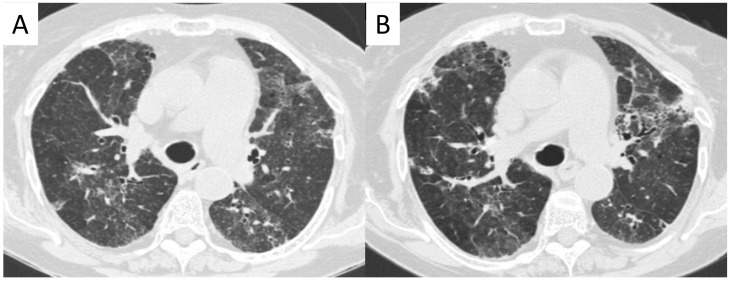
An 80-year-old female patient with granulomatosis with polyangiitis (GPA). HRCT shows irregular bronchial wall thickening, bronchial dilatation, and interstitial lung alterations with interlobular septal thickening and GGOs. It is possibly to appreciate areas of different attenuation of lung parenchyma, resembling the appearance of the so called “mosaic attenuation” (A). Follow-up CT (B), acquired one year later, confirms the presence of these alterations.
